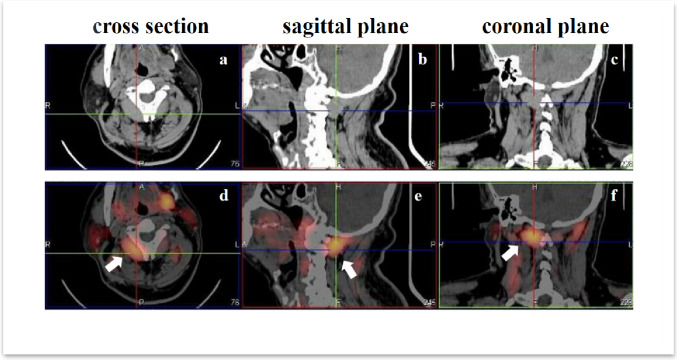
A case of ^99m^Tc-MIBI SPECT/CT muscle imaging. Note: Figures a, b, and c are CT scan images for muscle localization, and Figure d, e, and f are SPECT/CT fusion imaging images. The highly concentrated ^99m^Tc-MIBI region pointed by the arrow was observed at this level, integrating orientation of cross section, sagittal plane and coronal plane, and the region was in line with the right obliquus capitis inferior located in CT tomography images, and then the right obliquus capitis inferior was identified as one of the responsible muscles of the patient.
